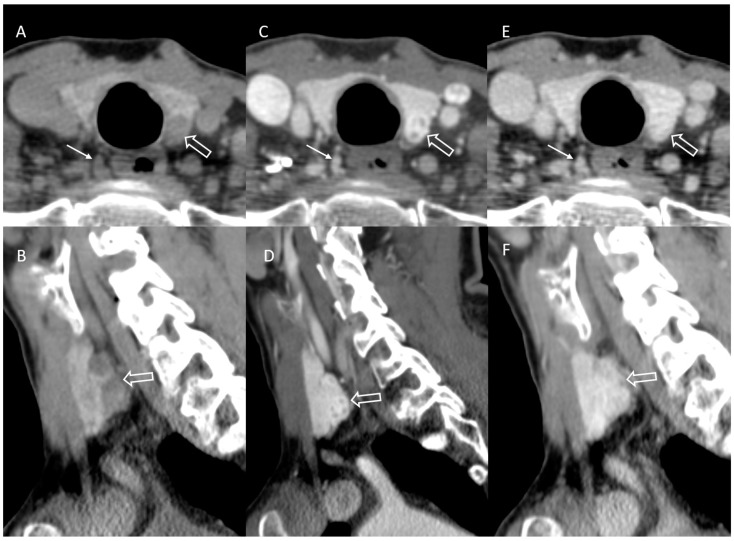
Incomplete initial outside and second read identification of parathyroid adenoma on CT. Outside neck CT was performed with the parathyroid protocol ((A,B) non-contrast, (C,D) arterial phase, (E,F) delayed phase) for the indication of primary hyperparathyroidism in a 67-year-old woman. Outside ultrasound and nuclear medicine sestamibi reports were negative, although the images were not available for comparison. The initial outside radiology impression and second read concurred and correctly identified a right paraesophageal parathyroid adenoma ((A,C,E) arrows). The initial report and second read both characterized the left thyroid lobe lesion as thyroid nodules ((A–E) open arrows). Bilateral neck exploration was performed, with frozen section and final pathology, confirming bilateral hypercellular parathyroid adenoma.
